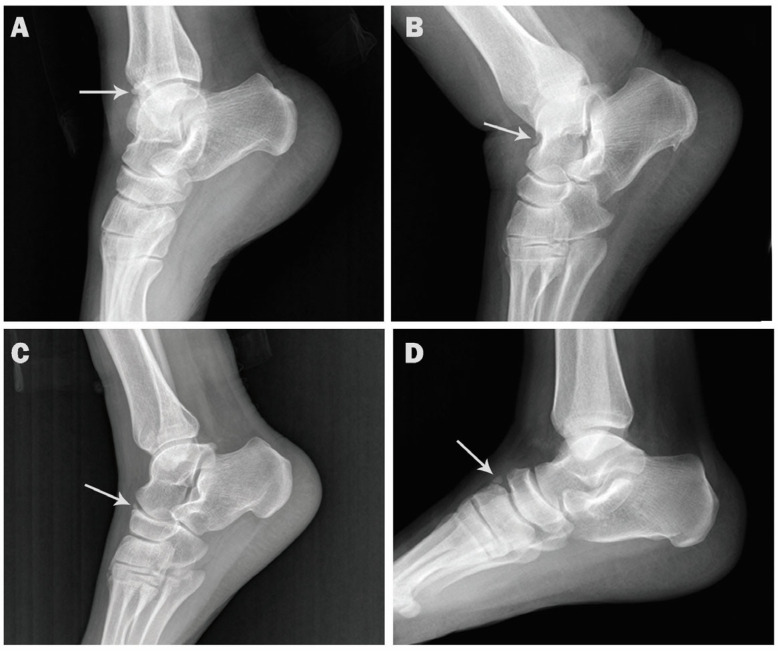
Lateral radiographs showing accessory ossicles (arrows). (A). Os talotibiale in a 38-year-old female. (B). Os supratalare in 47-year-old male. (C). Os supranaviculare in 51-year-old male. (D). Os infranaviculare in 25-year-old male.
